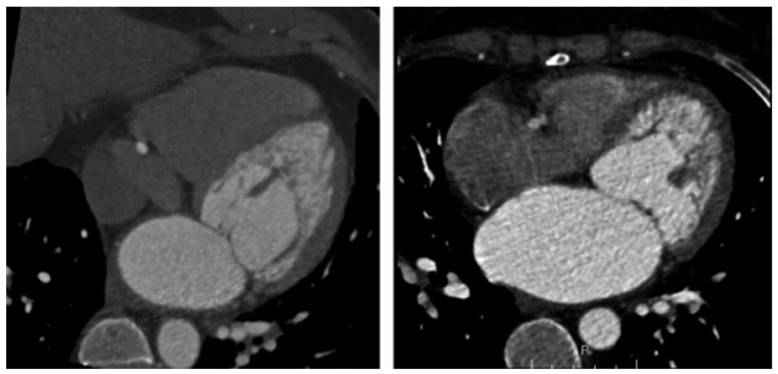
Axial view of cardiac computed tomography (CCT) imaging with coronary angiography showing LVNC. *Source: Rao, K., Bhaskaran, A., Choudhary, P., & Tan, T. C. (2020). The role of multimodality imaging in the diagnosis of left ventricular noncompaction. European Journal of Clinical Investigation, 50(9), e13254* [55].
